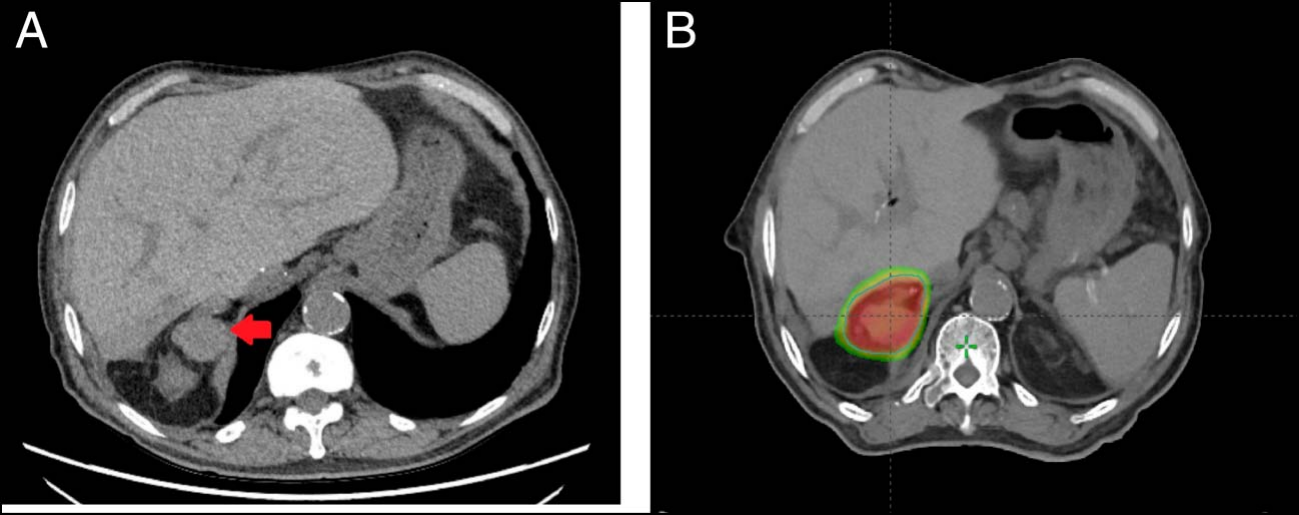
(A and B) Computed tomography of the chest showing the right adrenal mass (arrow).
